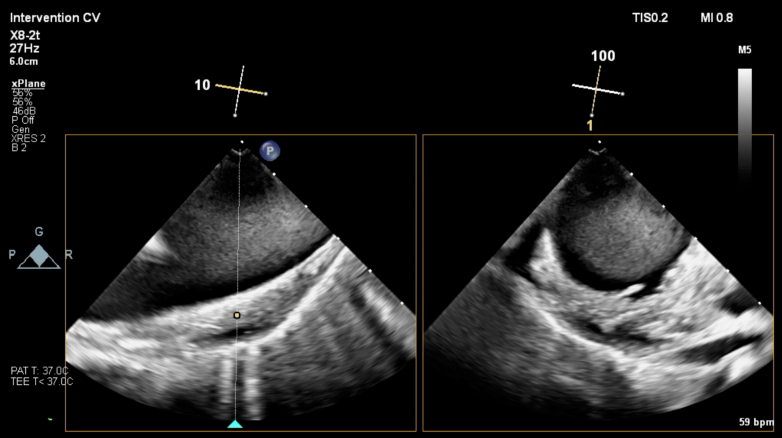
Intraprocedural Transesophageal Echocardiography Image Transesophageal echocardiography showing intramural hematoma after device dislodgement from the transverse aorta.
